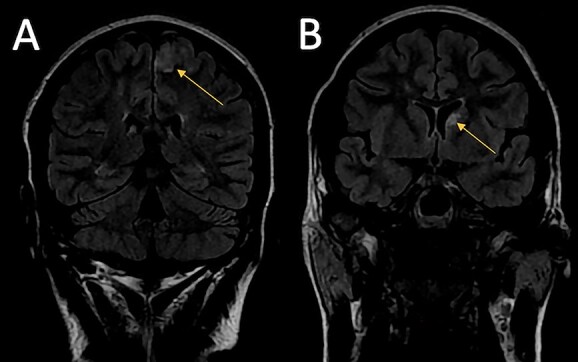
Images A and B are coronal views of the fluid attenuated inversion recovery (FLAIR) MRI sequence of the brain. Image A shows a high signal in the left paracentral lobule. Image B shows a high signal in the left caudate nucleus.
